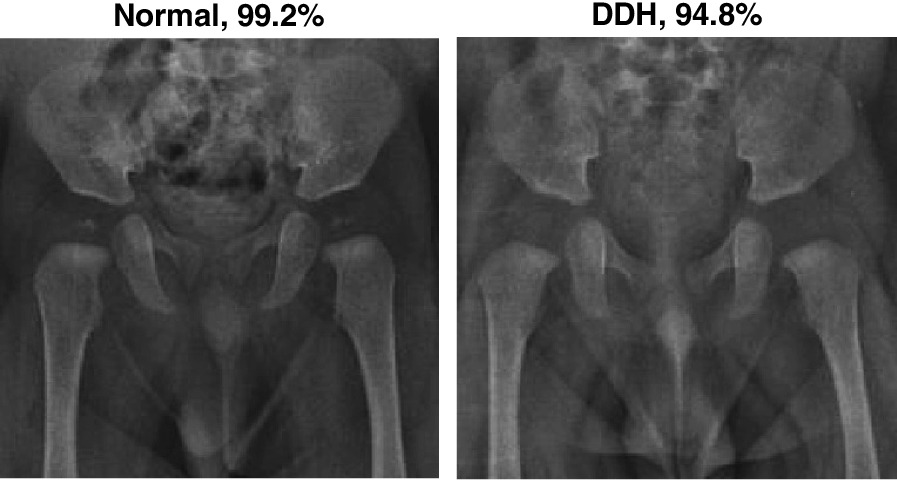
A sample of correctly classified X-ray images
